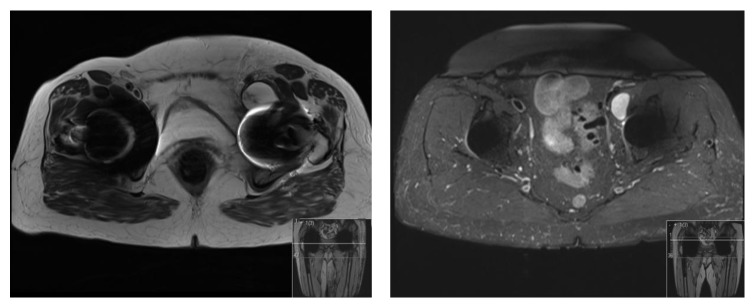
MARS MRI showing a left retroperitoneal cystic mass lesion (74 mm × 32 mm) noted anteriorly and extending into the iliopsoas bursa.
